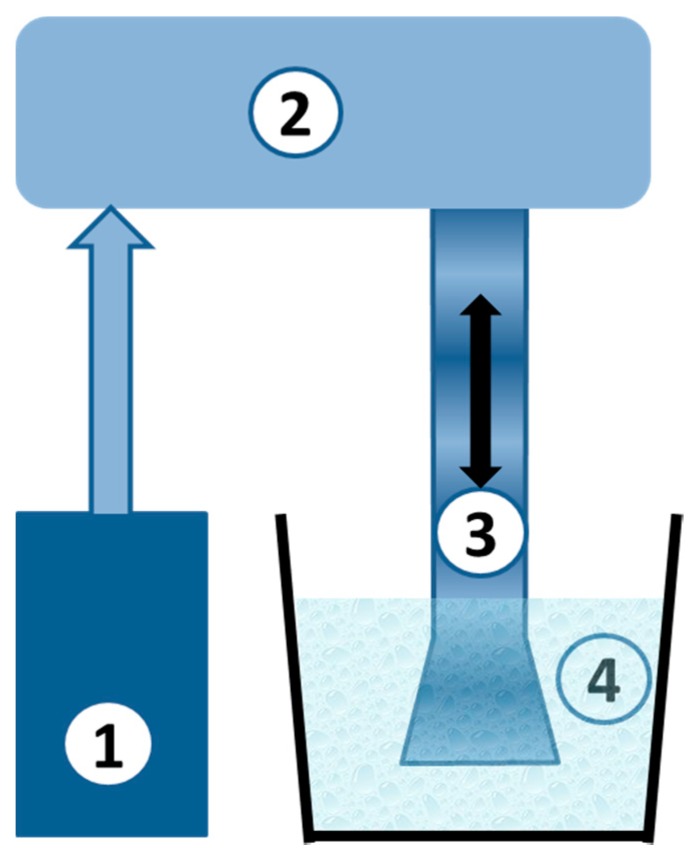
Scheme of the ultrasound device. 1—ultrasound generator, 2—magnetostrictive transducer, 3—waveguide, 4—vessel with water.
